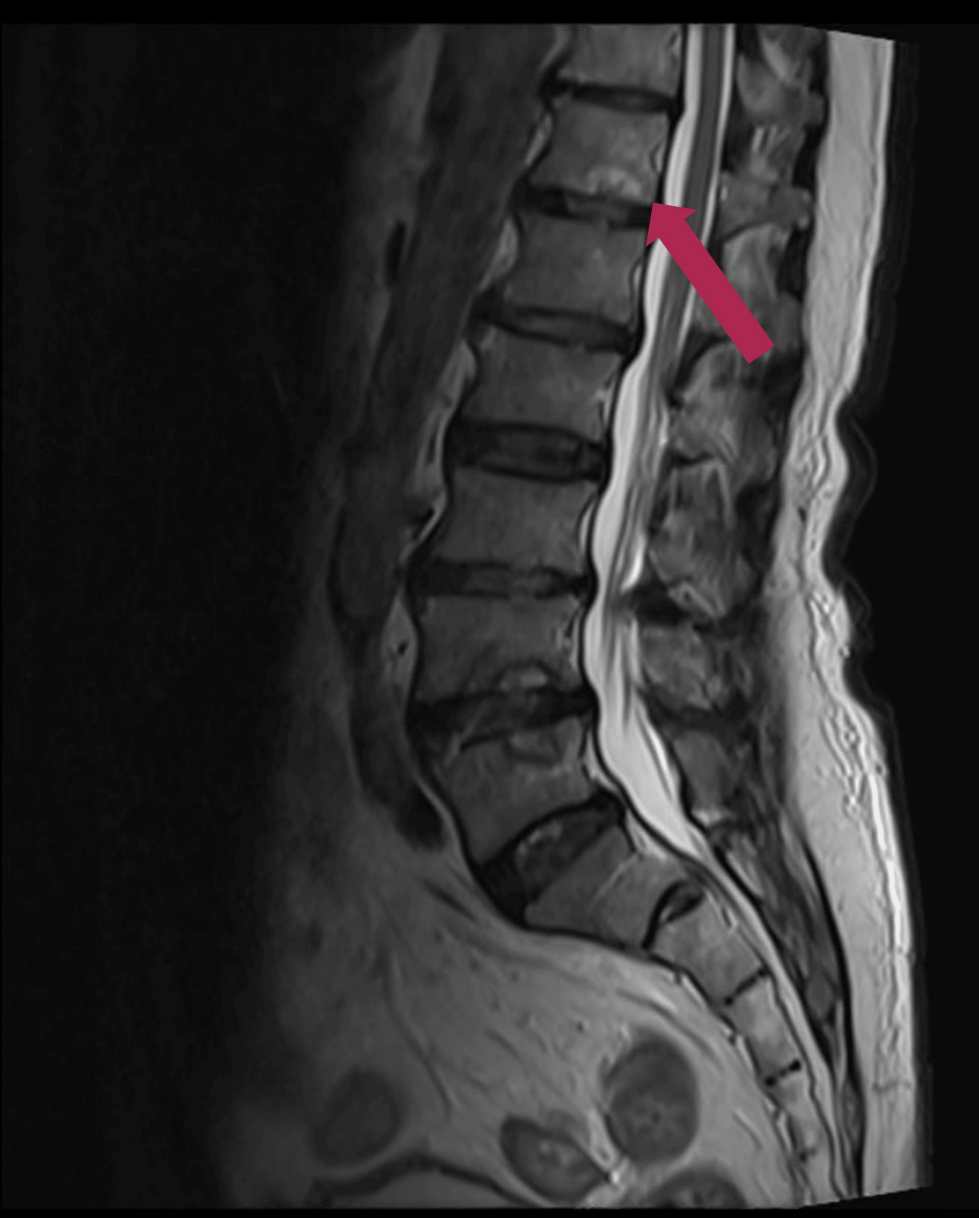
Follow-up lumbar spine MRI showing interval improvement of L4–L5 facet joint infection Follow-up MRI of the lumbar spine with and without contrast demonstrates interval improvement with mild residual inflammatory changes at the bilateral L4–L5 facet joints and no rim-enhancing fluid collection. No epidural collection or cauda equina compression is seen.
